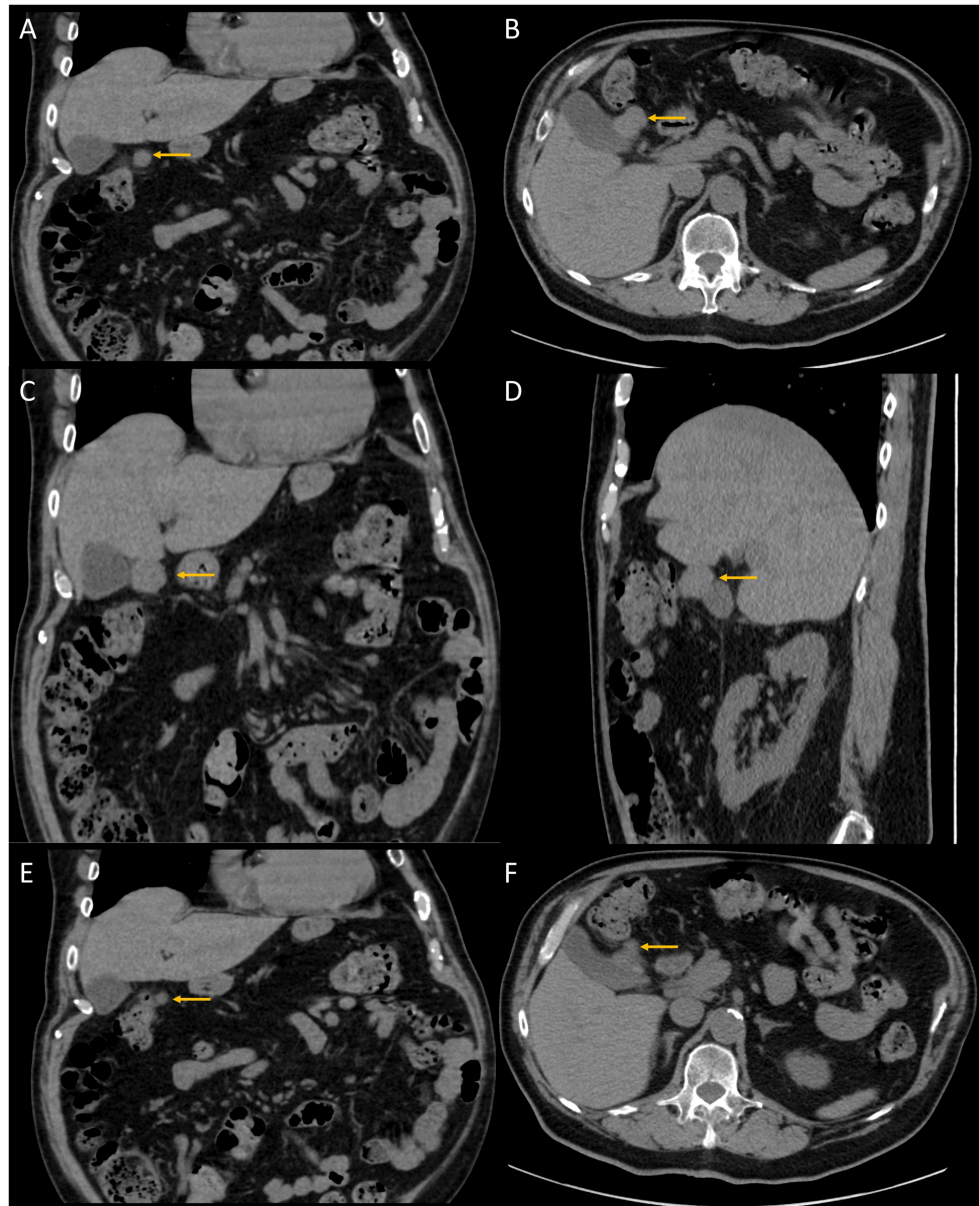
(A-D) Coronal and axial CT images demonstrate a loculated soft tissue mass arising epiphytically from the gallbladder wall and extending into the peritoneum (arrows in A and B). The fat plane between the mass and the inferior margin of the liver is obliterated in both coronal and sagittal views (C and D). (E–F) The mass has increased in size compared to the prior CT scan performed two years ago (arrows)
